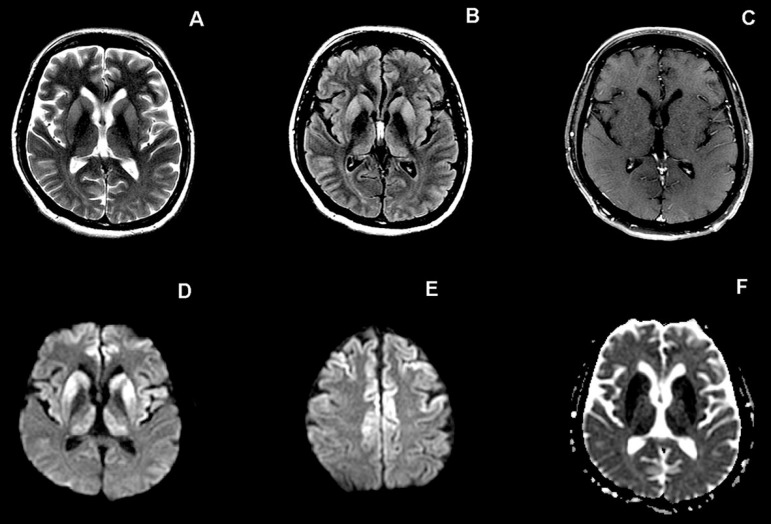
Case 1. Axial T2-weighted (A) and FLAIR (B) images: hyperintensity in basal
